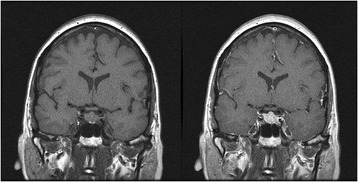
Pituitary MRI study. *Left*: T1 without contrast, *Right*: T1 with gadolinium contrast. Pituitary gland is symmetrically enlarged and enhances homogenously with gadolinium contrast
